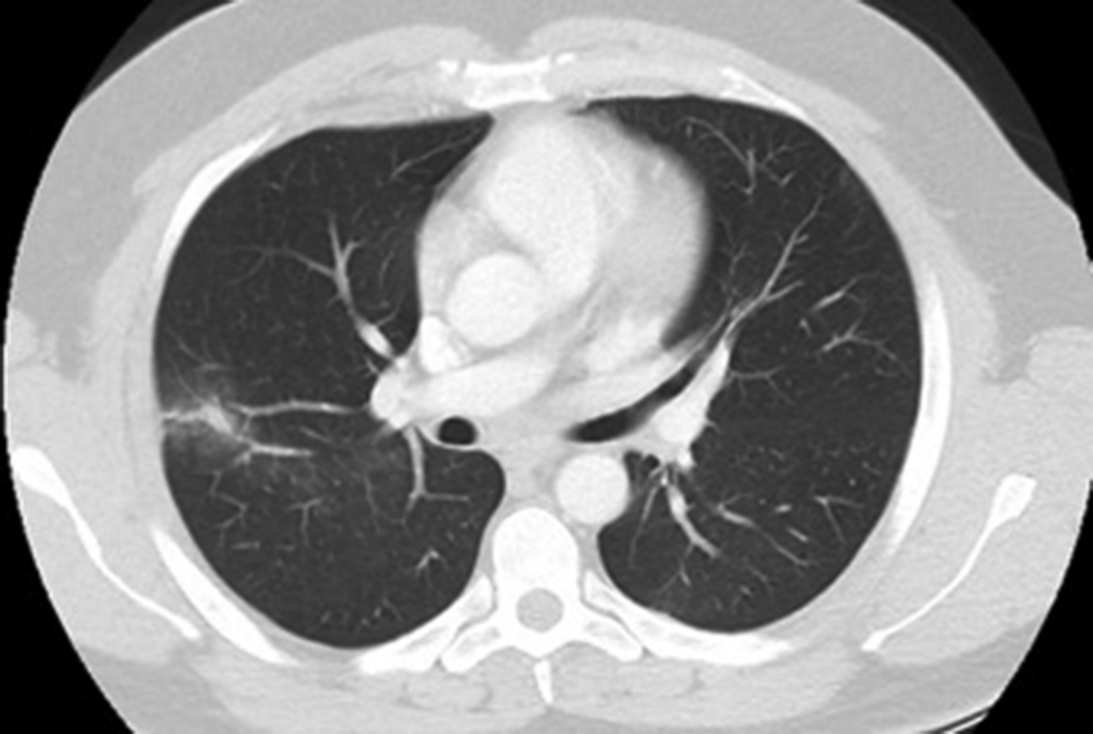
CT chest following extraction of cockroach.
